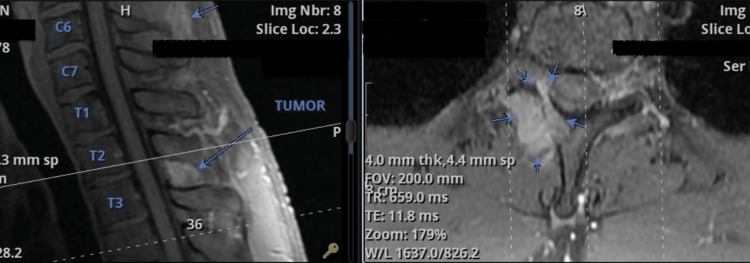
MRI (sagittal T1 with contrast) of the low cervical and thoracic segment of the spine showing an osteolytic lesion with its epicenter in the right lamina and inferior facet of the T2 vertebra. The lesion slightly invaded the right neural foramen, near the exiting right T2 nerve root, posteriorly to the spinous process of the C6 vertebra.
